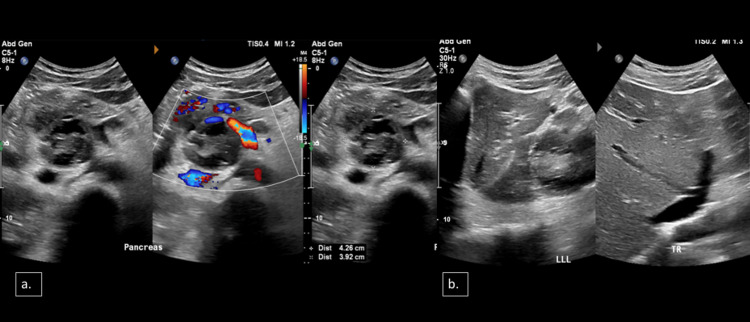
Selected axial ultrasound images of the upper abdomen (a) A heterogeneously echoic lesion with multiple cystic areas was noted in the region of the pancreatic head with peripheral vascularity (4.3 × 3.9 cm). (b) A mild degree of intrahepatic biliary radicles
